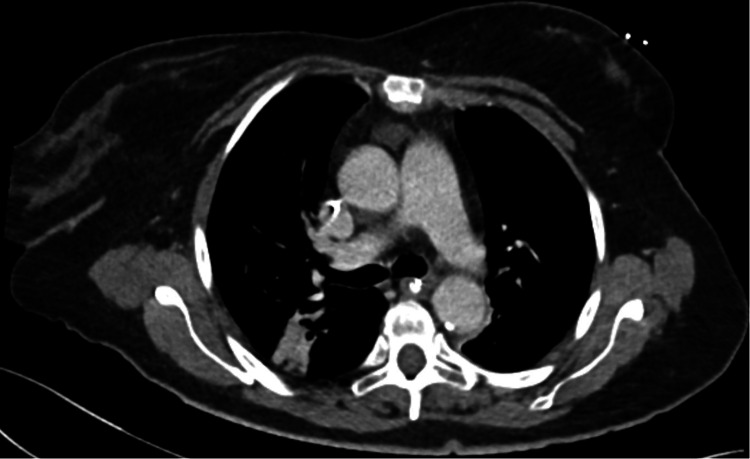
Chest CT to exclude thymoma
